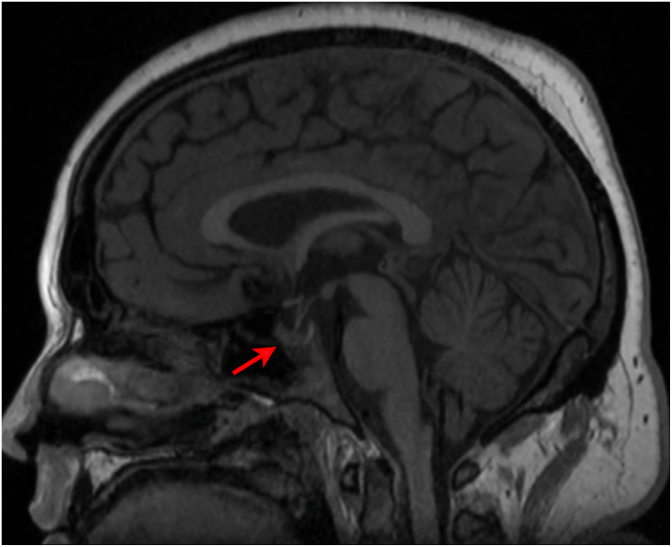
Sagittal T-1 weighted MR image showing a partially empty sella (arrow) at the time of clinical presentation, suggesting a history of chronic elevation of ICP.
